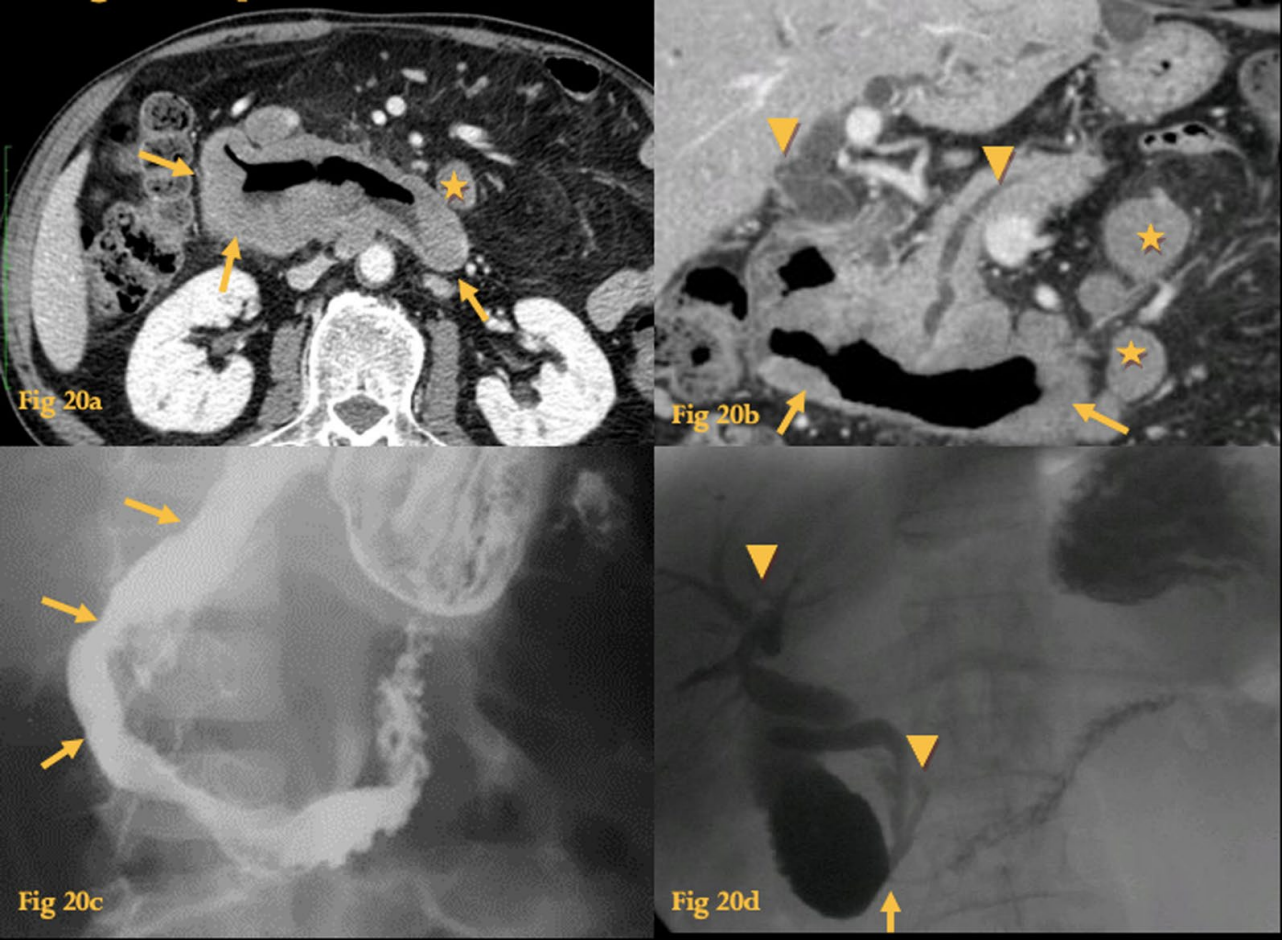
Lymphoma. Contrast-enhanced CT (a, b) shows homogeneous and asymmetric thickening of the duodenal wall with mild dilatation of the lumen (arrows); there is biliary and Wirsung duct dilatation (arrowheads); paraduodenal adenopathy is also evident (asterisks). UGIS of the same patient (c) shows loss of the normal mucosal pattern of folds at the descending duodenum (arrows). UGIS some months after the first UGIS, while on chemotherapy (d) shows abrupt luminal narrowing at the horizontal duodenum (arrow), with dilatation of the proximal duodenal segments. Because of increased pressure at the stenosis, there was contrast reflux to the biliary ducts and Wirsung duct (arrowheads)
